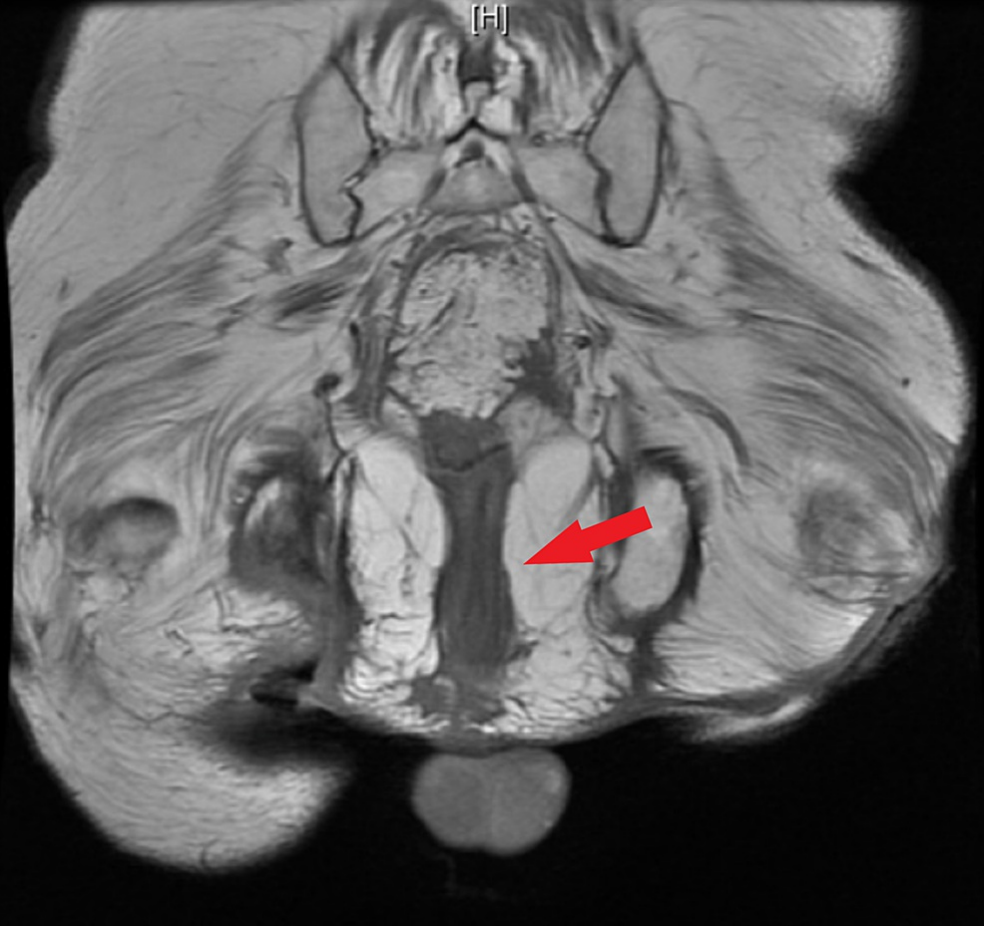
Coronal view of pelvic MRI. Red arrow indicates the rectal stump without any visible abscess or fistula. MRI, magnetic resonance imaging
